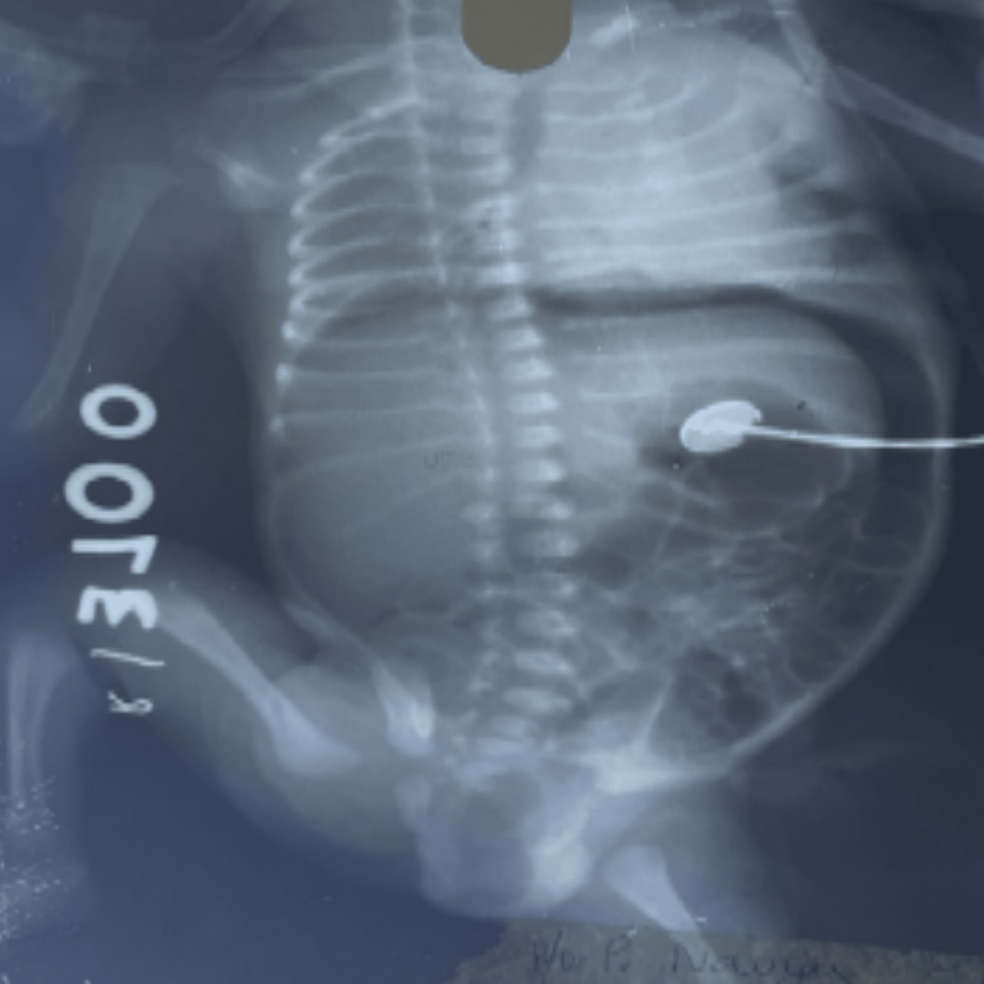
Abdominal radiograph in supine view showing pneumoperitoneum
